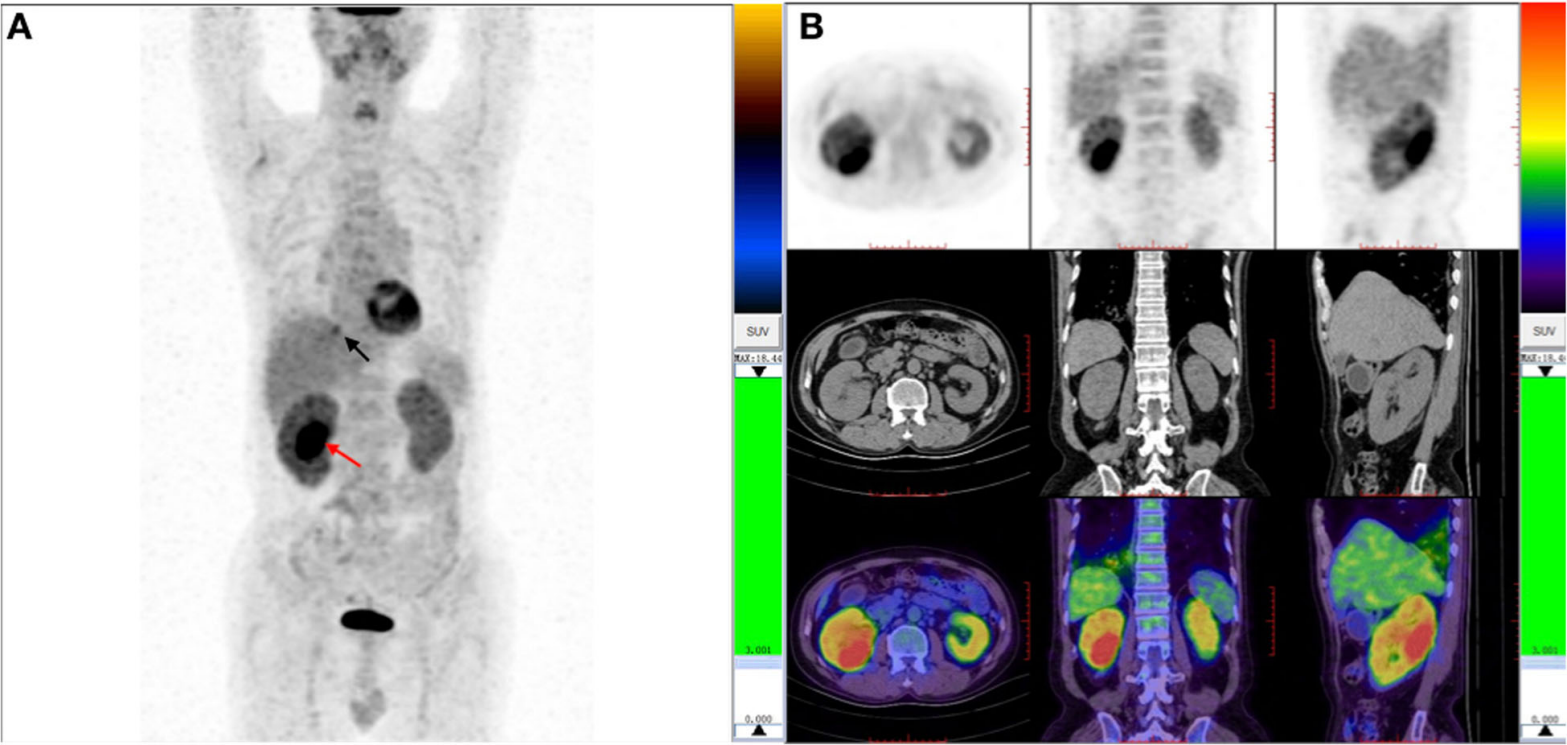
18F-FDG PET / CT images: There were bilateral plump kidneys and FDG slightly avid (SUVmax 4.6). There was no obvious abnormal density change in the local mass of the right dorsal kidney, but FDG uptake was significantly increased (SUVmax 11.1) and the concentration range was about 28 mm × 42 mm × 50 mm (AP × RL × SI) (red arrow). The interlobular space of the lower lobe of both lungs were thickened and changed in grid-like manner, with the posterior basal segment and the outer basal segment of the right lower lobe as the most obviously. The FDG uptake increased, and SUVmax was 5.1 (black arrow)
